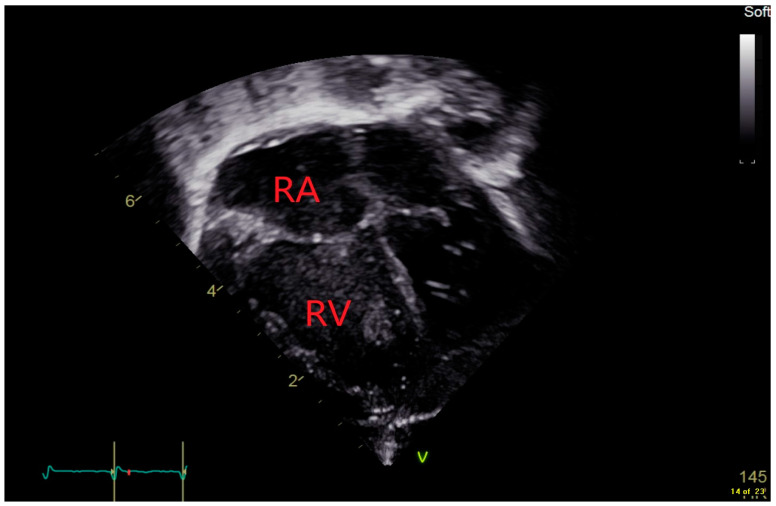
Apical 4-chamber view showing the dilated right atrium (RA) and right ventricle (RV).
